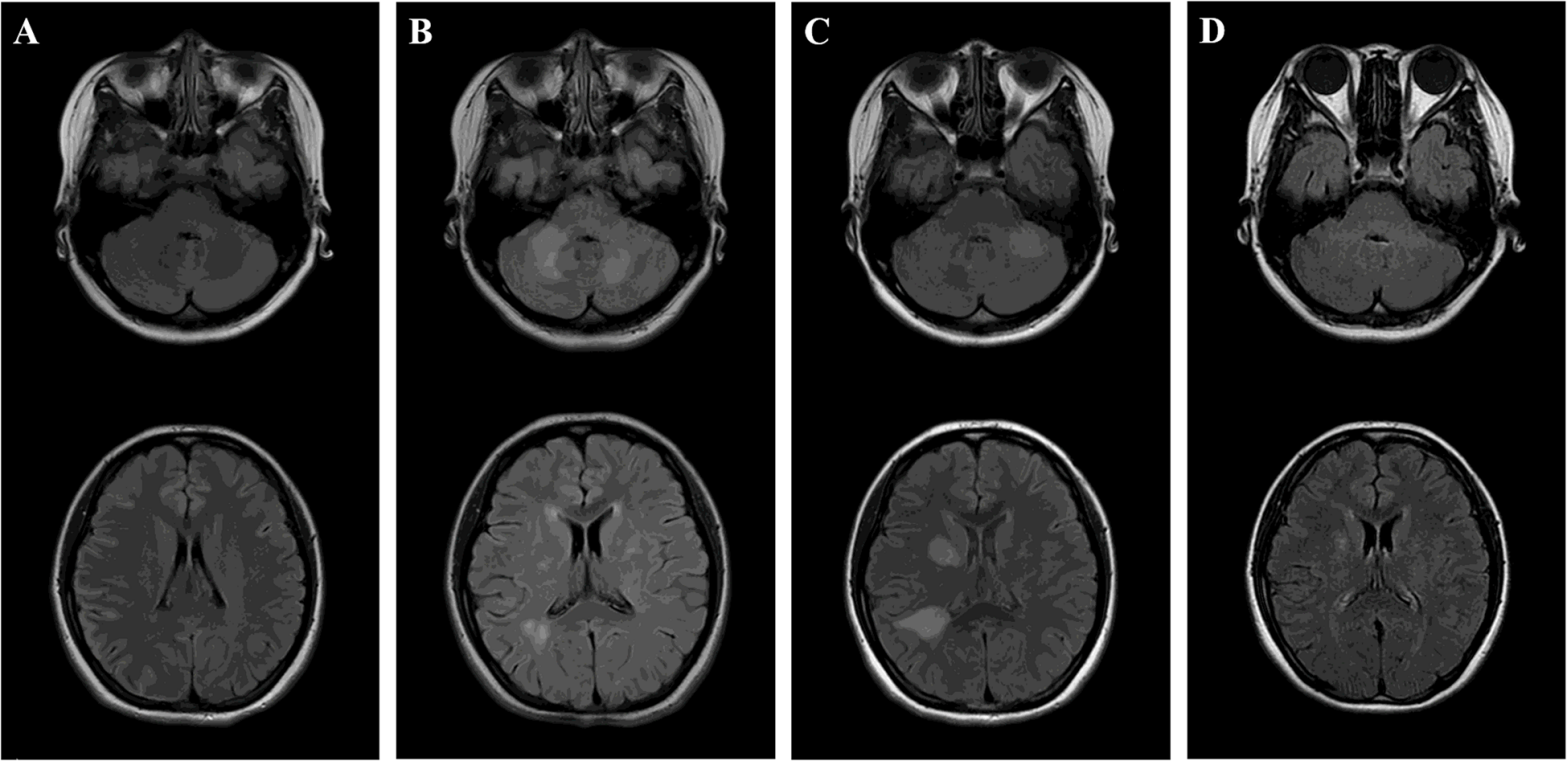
Serial axial T2 FLAIR images at presentation and follow-up. Initial axial brain T2 FLAIR image (A) revealed no obvious abnormalities in brain parenchyma. Repeated MRI after 3 months (B) showed asymmetric hyperintense signal change of the cerebellum, corona radiata, frontal and parietal white matter. MRI at 4 months from presentation (C) showed a partial reduction in lesions, but enlarged areas in left cerebellum and right parietal white matter, and new lesion in the region of the right ependyma. MRI at 6 months from presentation (D) revealed obviously resolution of abnormalities
